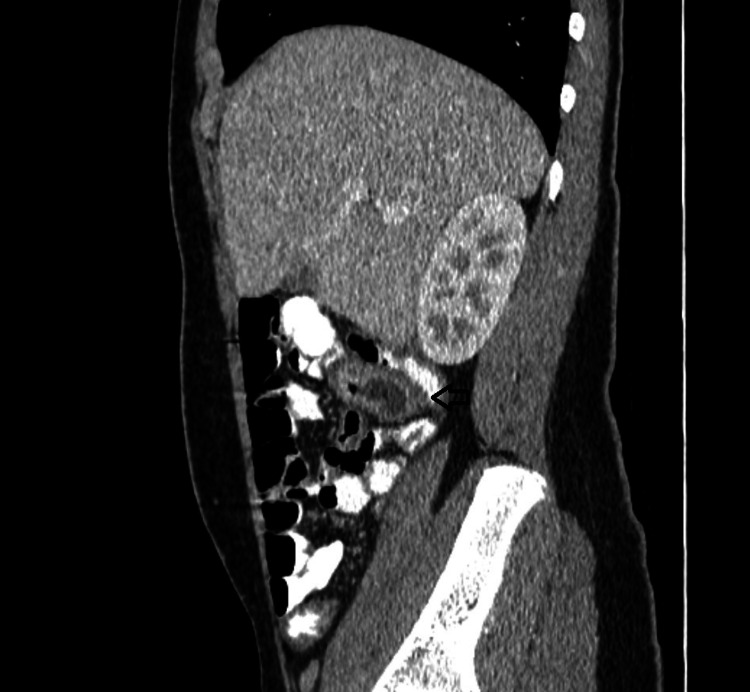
Enhanced CT with oral contrast, sagittal view showing the communication of Meckel’s diverticulum with the small intestine
